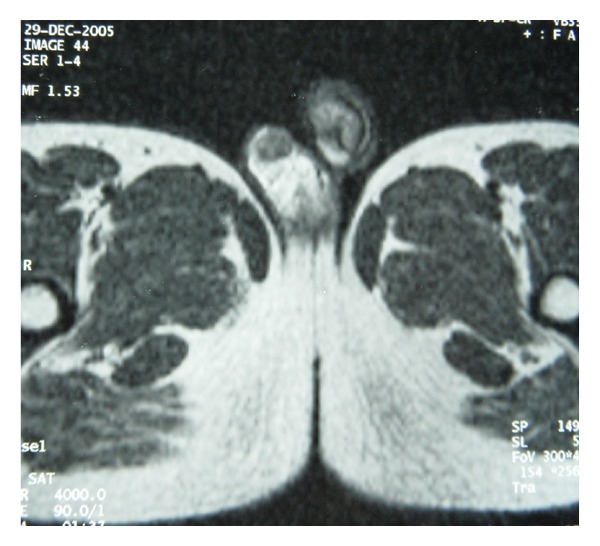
Testicular T2-weighted images showing hypointense lesion in the right epididymis, with central areas of high signal intensity.
